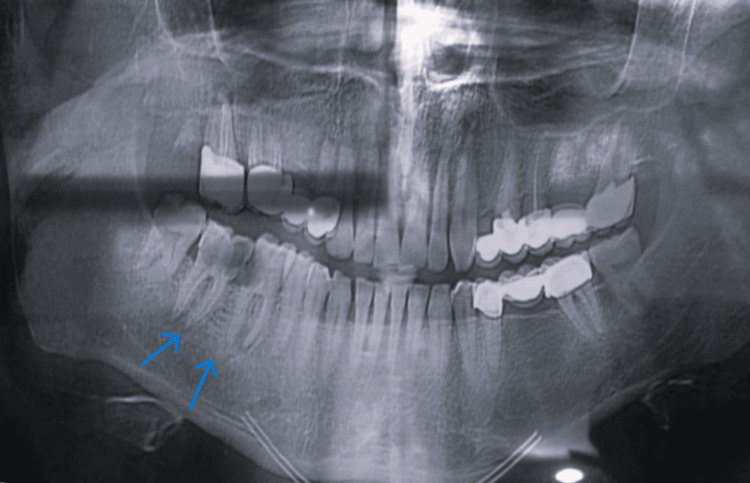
Panoramic radiograph The image shows decayed teeth 46 and 47 and abscess lesions (blue arrows).
